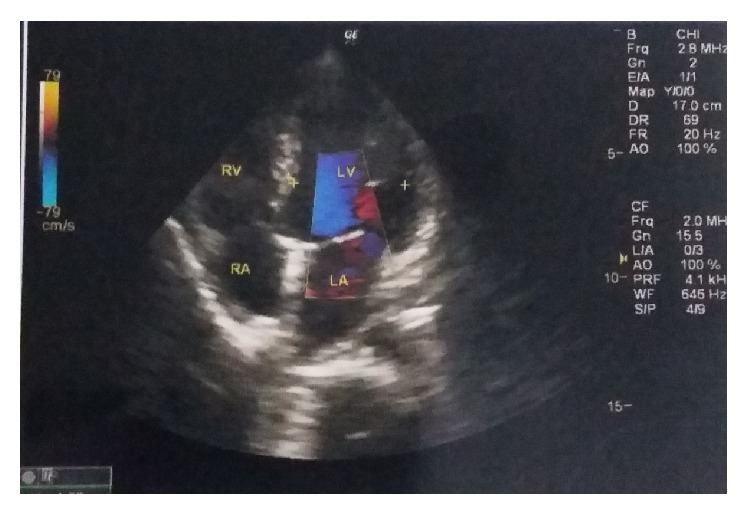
Echocardiography of the patient.
